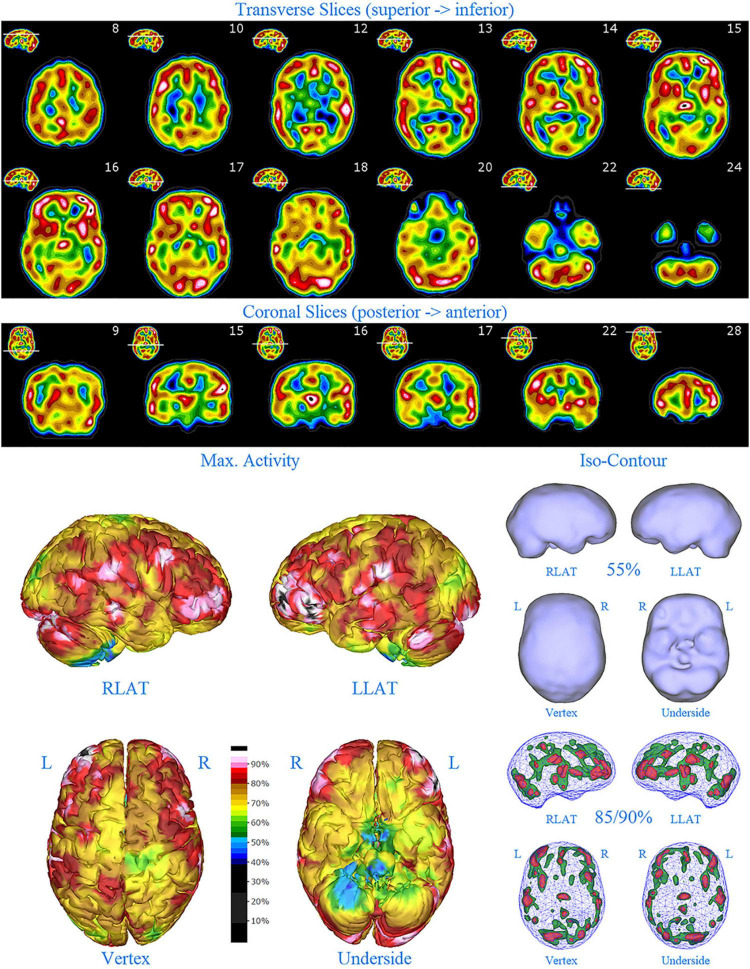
Second perfusion SPECT scan of Patient E. Arrangement, color scales, and views are identical to Figure 1. Anatomical structures such as the thalamus are positioned similarly to the scan shown in Figure 2. Labeling was eliminated to avoid obscuring the scan details. Perfusion of the thalamus is increased and asymmetrical. Areas of increased perfusion are considerably more prominent compared to the first scan, particularly in the left frontal cortex.
